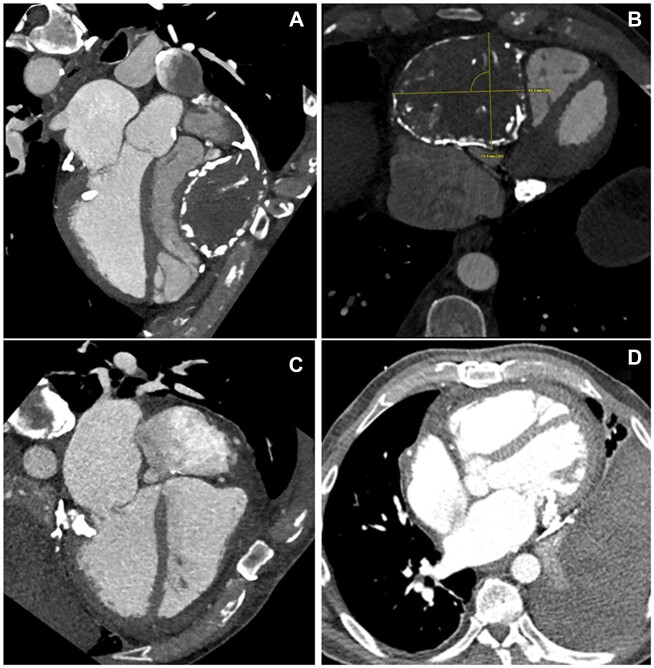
Preoperative CECT chest showing calcified pericardial cyst significantly compressing the tricuspid annulus, right atrium and right ventricle (A and B). Postoperative CT chest showing the complete removal of the pericardial cyst and relieved right atrial and right ventricle compression (C and D).
